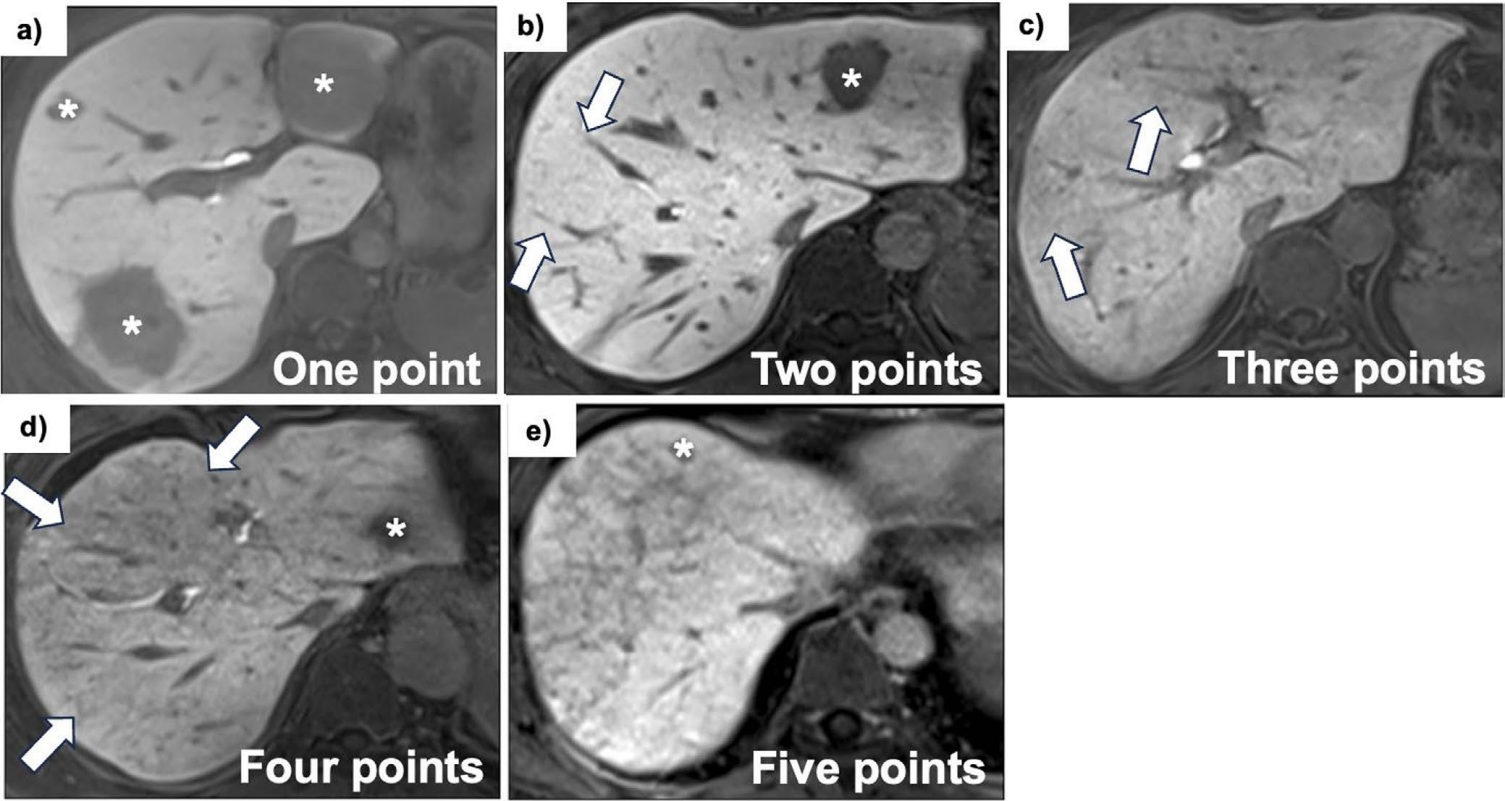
Representative hepatobiliary‐phase images from EOB‐MRI demonstrating the range of SOS severity, classified according to the degree of reticular hypointensity and the corresponding confidence levels: (a) Homogeneous high signal intensity across the liver parenchyma, indicating absence of SOS (confidence level = 1); (b) Faint reticular hypointensity (arrows) detectable in a limited number of sections, but the findings remain equivocal (confidence level = 2); (c) Fine reticular hypointensity localized to restricted areas (arrows), suggestive of mild SOS (confidence level = 3); (d) More pronounced reticulations distributed diffusely throughout all sections (arrows), consistent with moderate SOS (confidence level = 4); (e) Marked and extensive reticular hypointensity throughout the liver parenchyma, indicative of severe SOS (confidence level = 5). Hypointense nodular lesions indicated by asterisks (*) correspond to metastatic tumors, clearly distinguishable from SOS‐related alterations. EOB‐MRI, ethoxybenzyl‐diethylenetriamine pentaacetic acid‐enhanced magnetic resonance imaging; SOS, sinusoidal obstruction syndrome.
